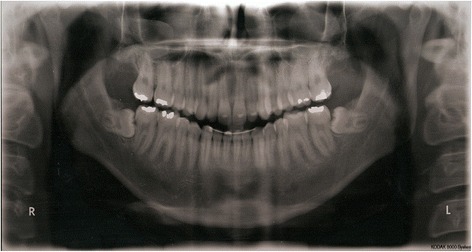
Panoramic radiograph of the same patient showing the M3 development along 5 years (T1)
